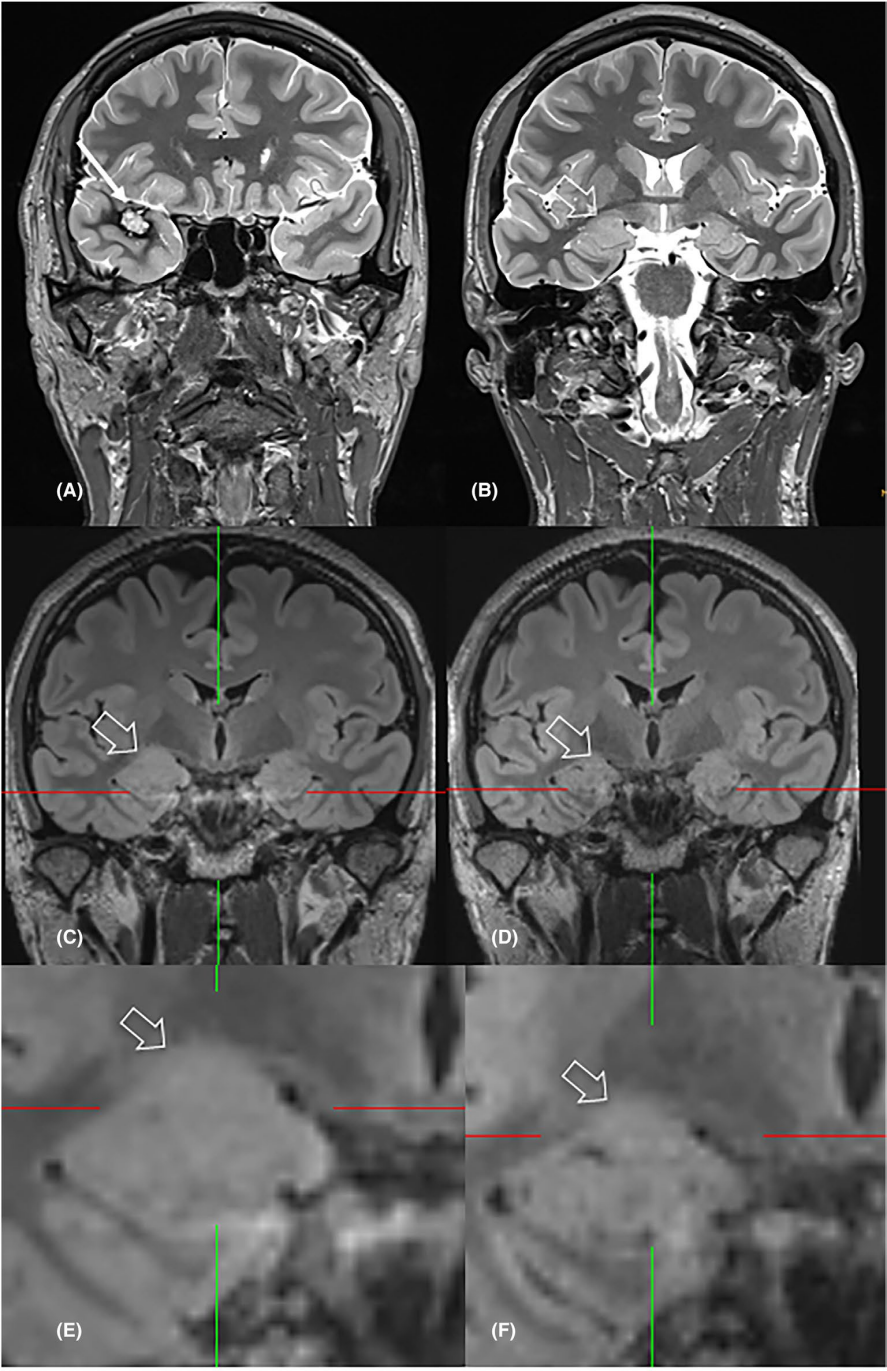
A 40 year‐old man (#32) presented with bilateral tonic clonic seizures. MRI revealed a cavernoma of the right superior temporal gyrus (A, C: Arrow) and right‐sided amygdala enlargement (B, D: Hollow arrow). Three months after cavernoma resection (E: Arrow) the patient was seizure‐free and amygdala enlargement had regressed (F: Hollow arrow).
